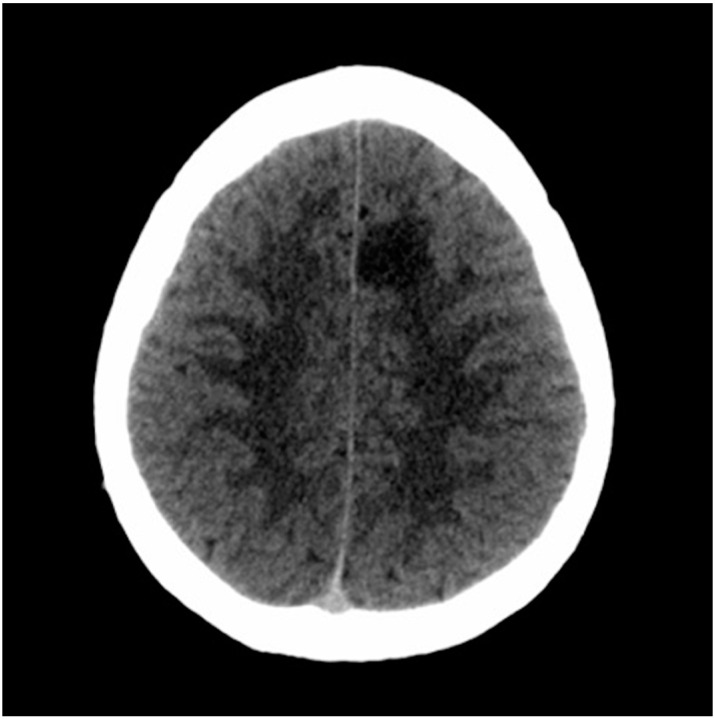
Computed tomography scan of the brain. The hypodense lesion in the medial left frontal lobe could be compatible, among other possibilities, with subacute ischemic stroke in the vascular territory of the left anterior cerebral artery.
